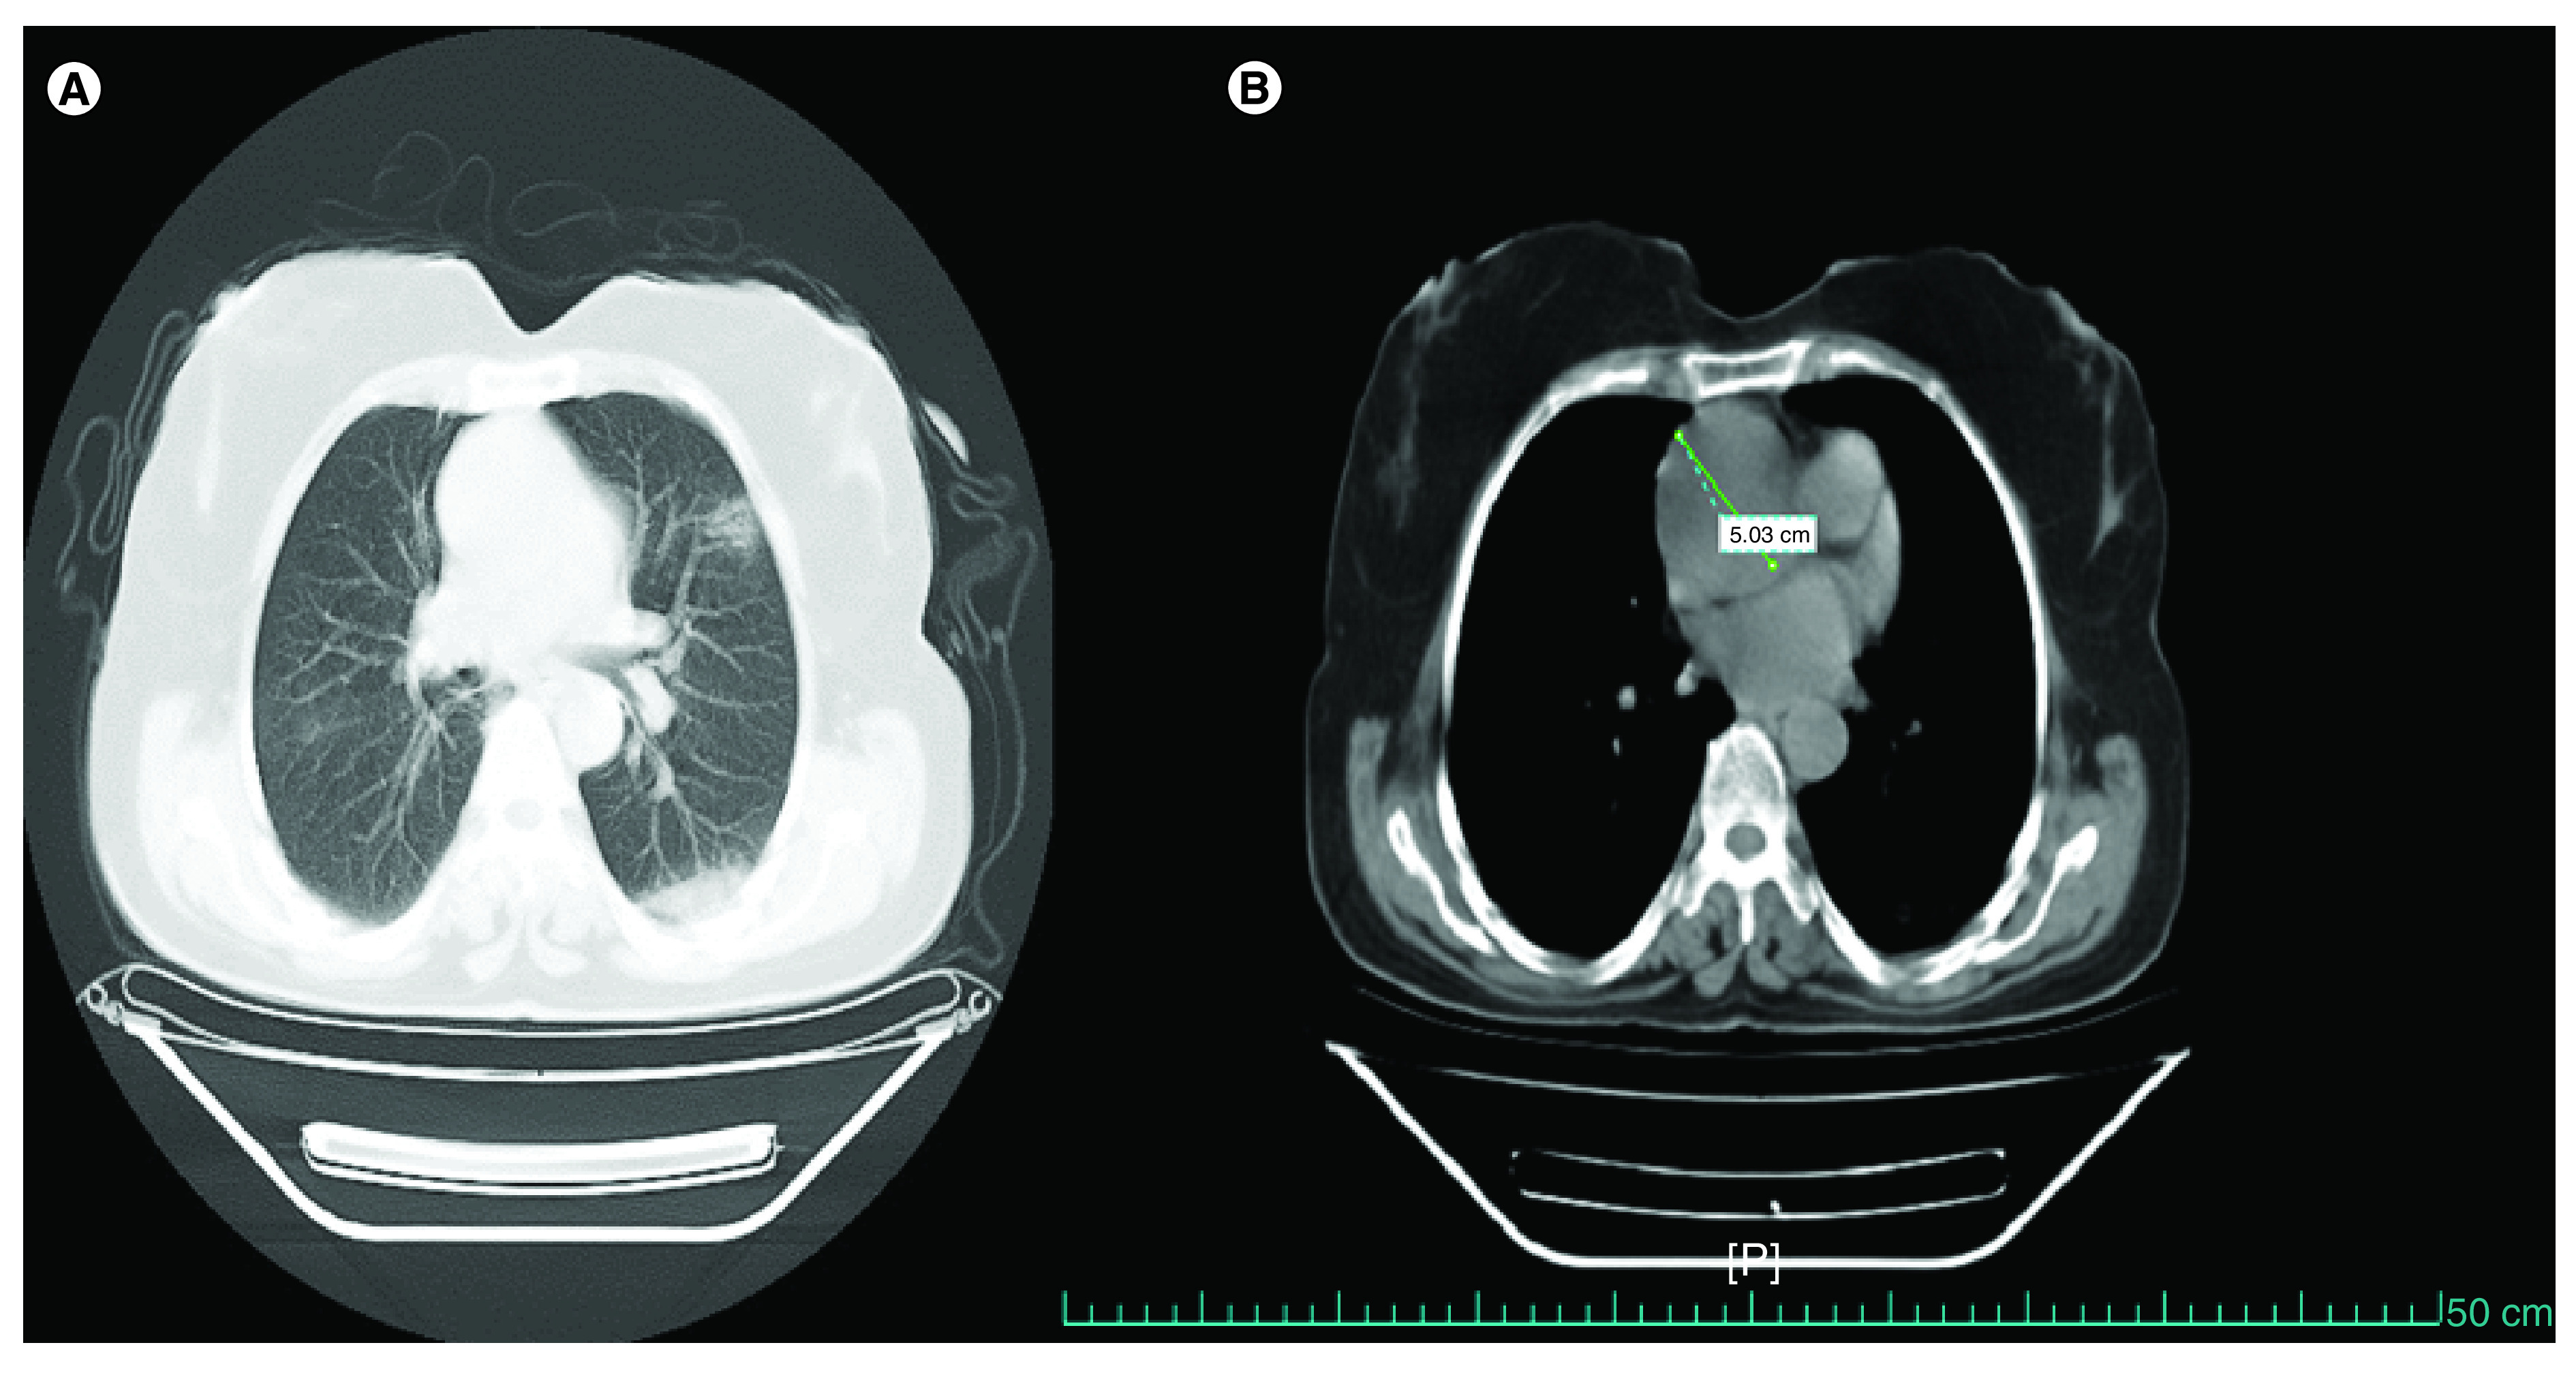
Chest computed tomography. (A) Patchy ground glass opacities and consolidation and (B) dilated aortic root up to 5 cm.
